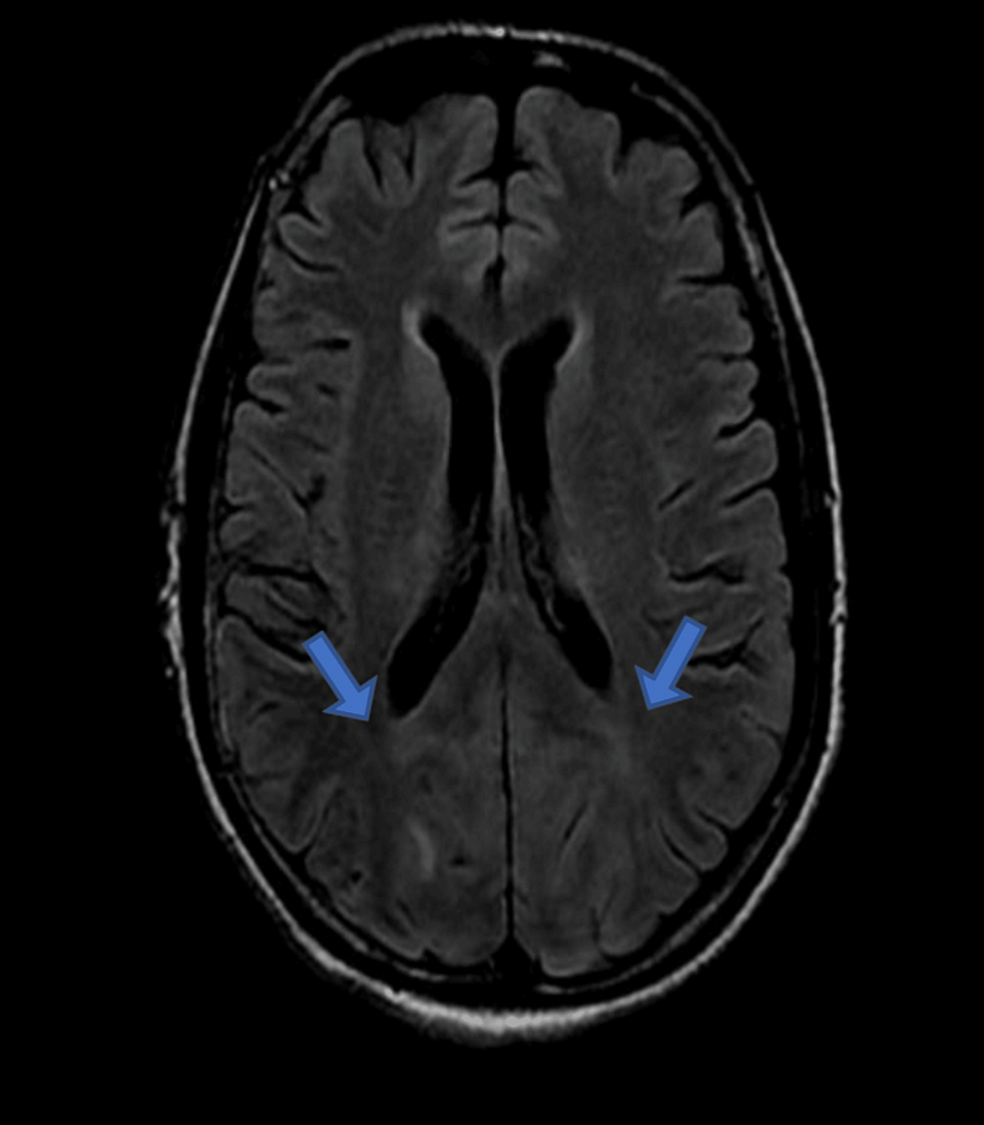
MRI brain coronal section showing hyperintensities in FLAIR sequence in the bilateral parieto-occipital lobe extending in asymmetric fashion to the bilateral cerebellum, brainstem, and thalami predominantly involving the white matter FLAIR: fluid-attenuated inversion recovery
